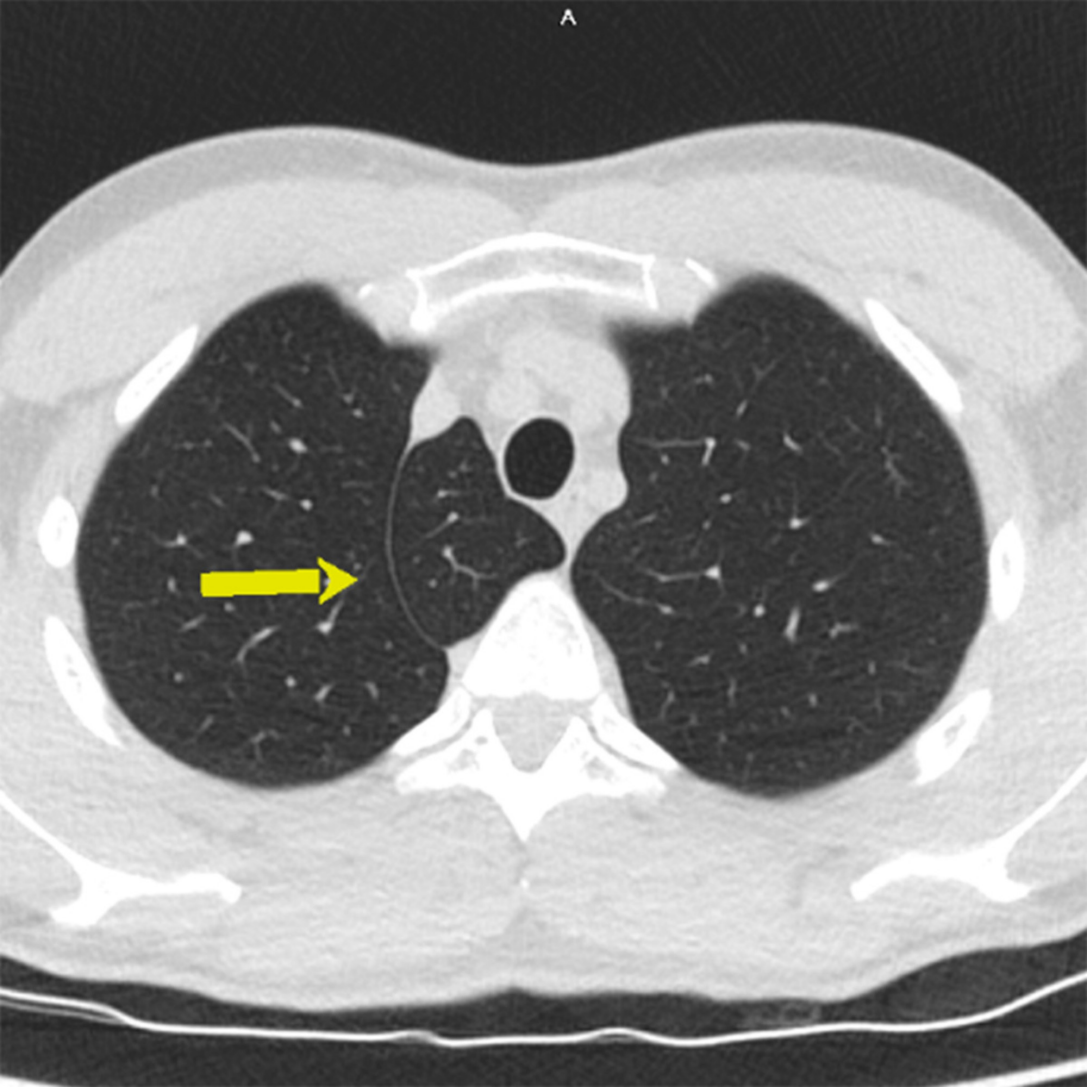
Computed tomography (CT) of the chest - axial image; azygos lobe (arrow)
